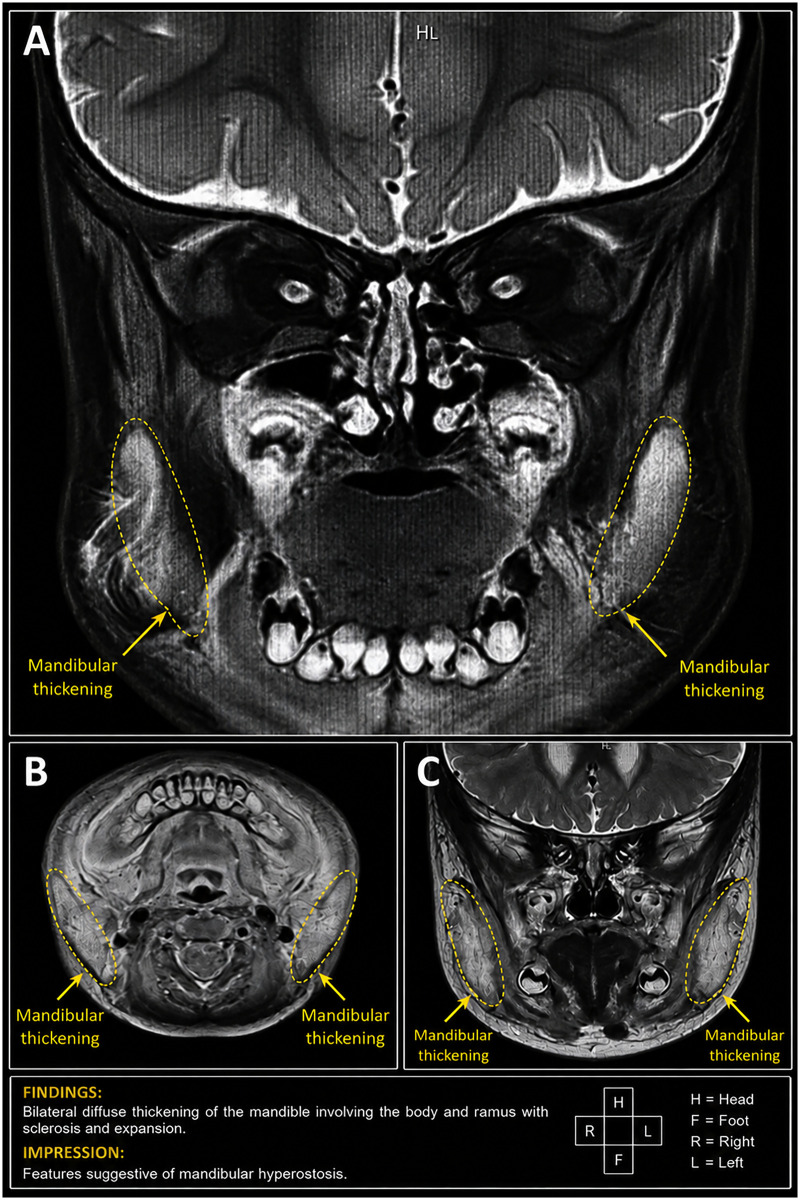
Multi-modal imaging assessment of a pediatric case of infantile cortical hyperostosis (Caffey disease) (A) coronal T2-weighted MRI demonstrating multiple unerupted tooth follicles within the maxillary and mandibular structures; (B) posterior coronal T2-weighted MRI highlighting diffuse soft tissue hyperintensity suggestive of masseteric myositis and developing permanent follicles; (C) axial T2-weighted MRI showcasing the overall dental arch form and relationship to unerupted maxillary dentition; three-dimensional computed tomography (CT) scan demonstrating diffuse cortical thickening of the entire mandible with pathognomonic periosteal reaction.
